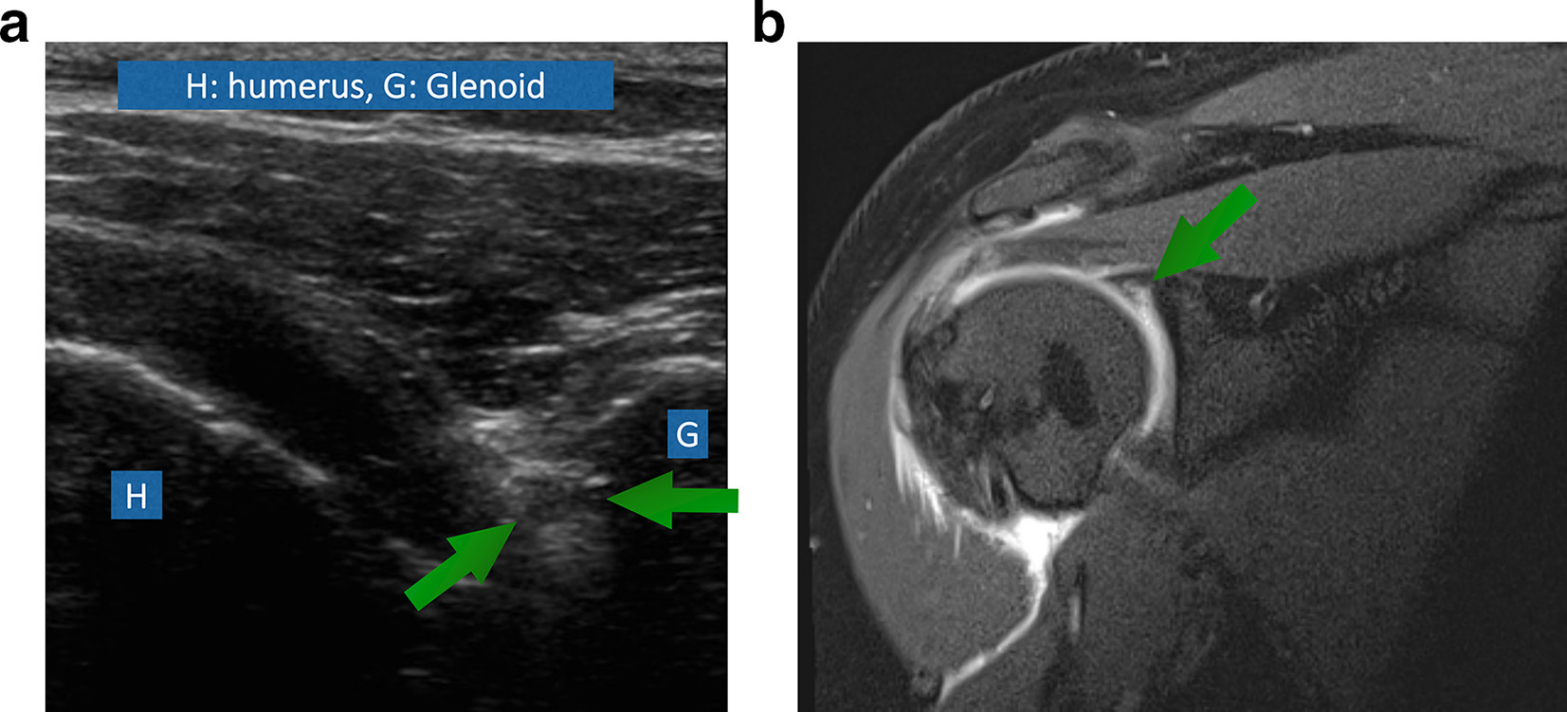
(A) A 38-year-old female patient came with recurrent subluxations. Ultrasound (Figure 6A) shows hypoechoic labral tear extending perpendicularly to its attachment to the bony glenoid in keeping with Type 3 SLAP lesion. Similar findings are seen on MRI (Figure 6B). Arrows depict the tear.
